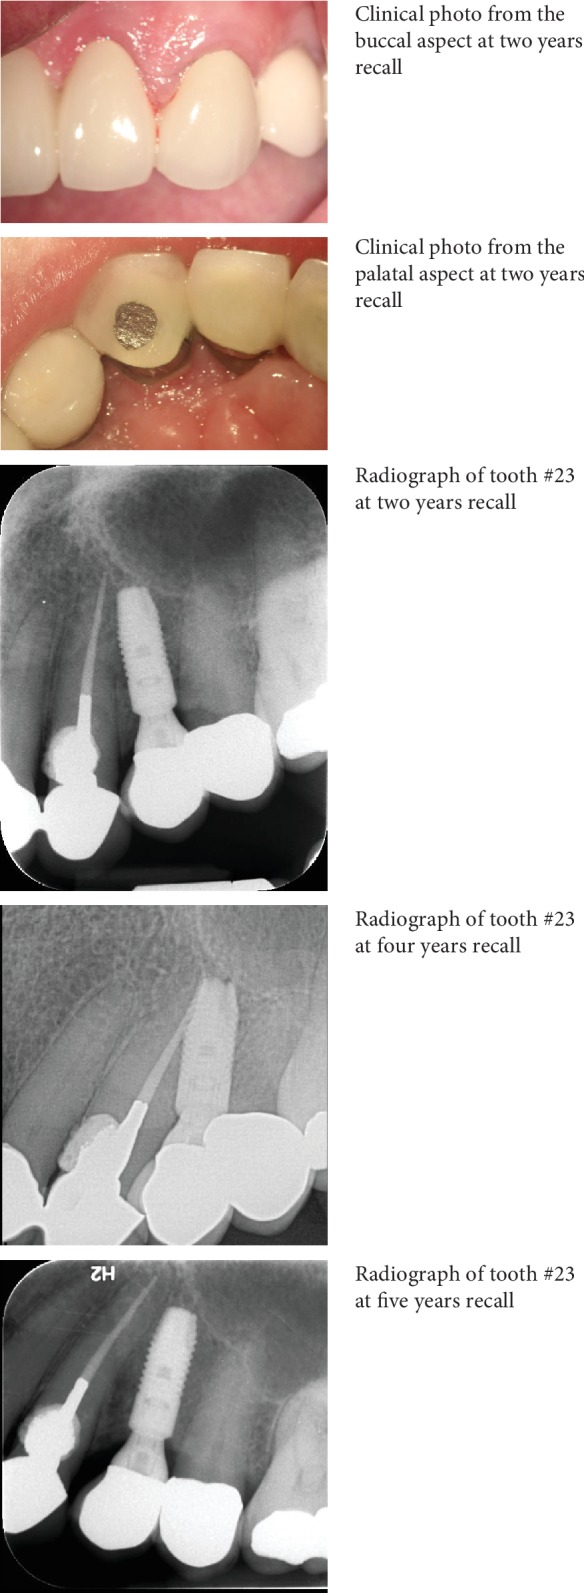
Recall radiographs and clinical photos for tooth #23.
